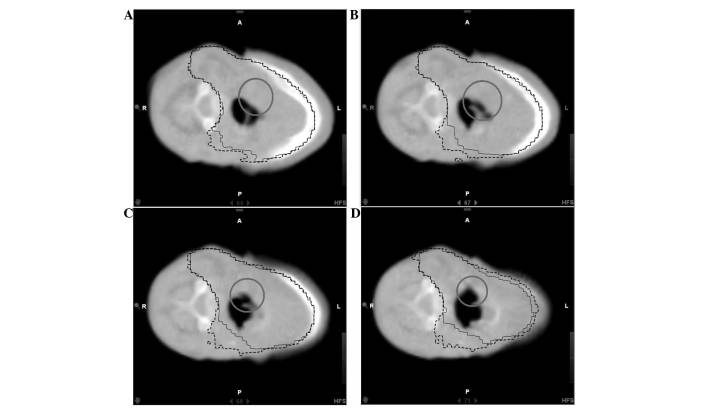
Planned and actual delivered dose. The solid line corresponds to the planned 95% isodose (60.8 Gy) and the dashed line to the actual delivered 95% isodose. The circle corresponds to the tumor recurrence site. Depicted are CT slices at different levels: (A) slice number 65; (B) slice number 67; (C) CT slice number 69; (D) CT slice number 71.
